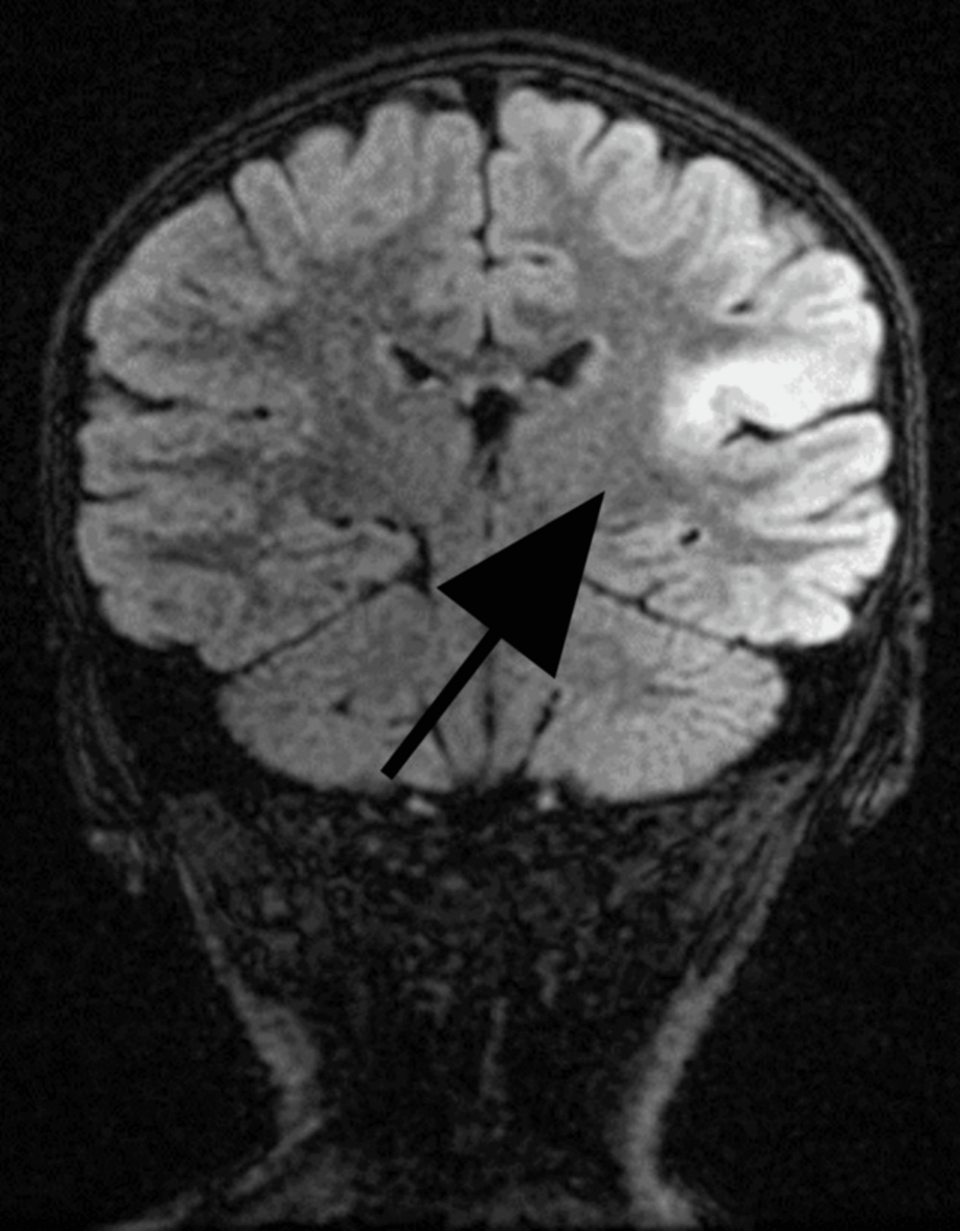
Brain MRI (coronal FLAIR) Cortical thickening and blurring of the grey-white junction in the left parietal cortex and T2/FLAIR hyperintensity of subcortical white matter with a transmantle sign MRI, magnetic resonance imaging; FLAIR, fluid-attenuated inversion recovery
